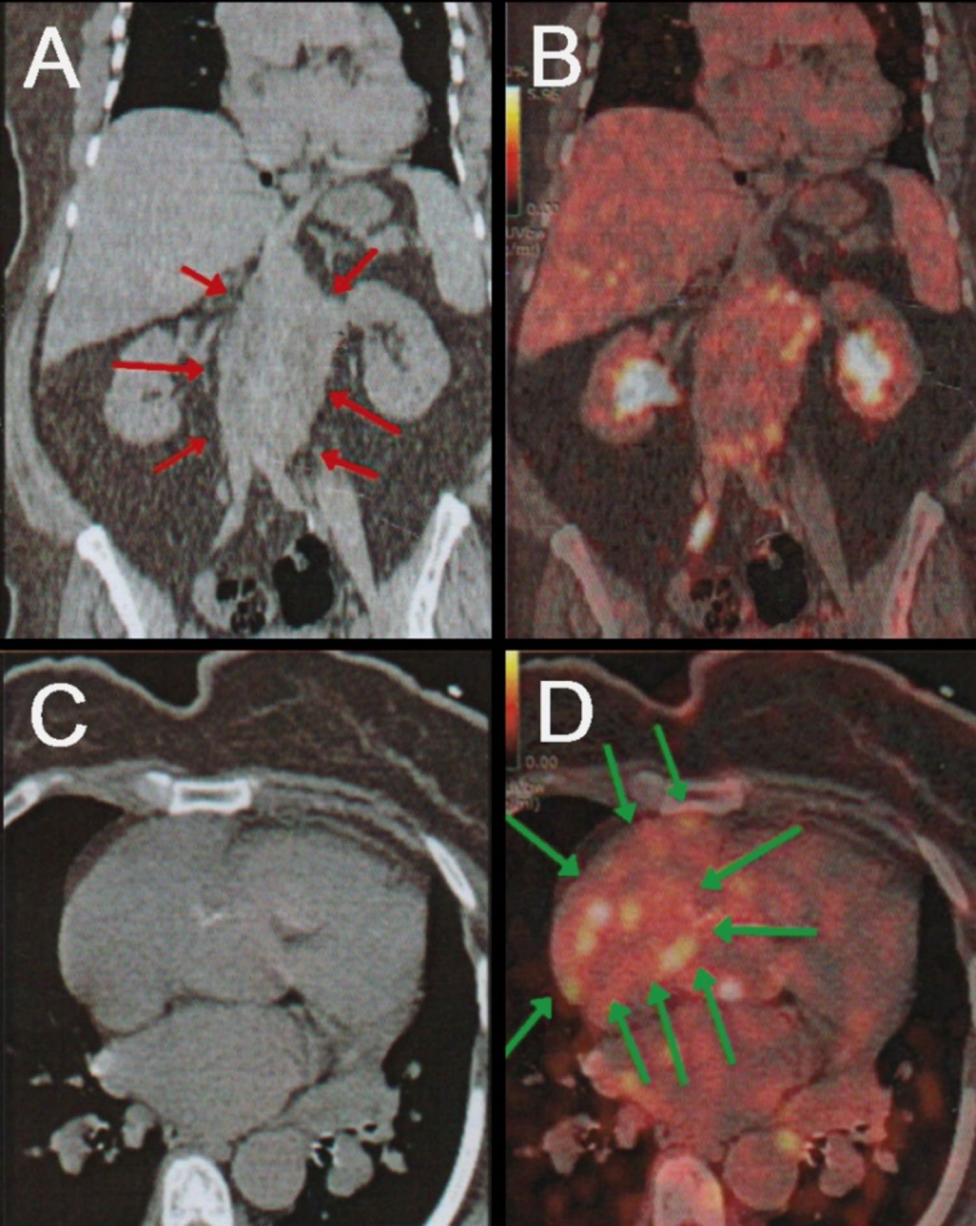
A 48-year-old female with IgG4-related disease. A Coronal non-contrast-enhanced computerized tomography (CT) and B coronal 18 fluorodeoxyglucose (FDG)-positron emission tomography (PET)/CT fusion images show 18 FDG-avid retroperitoneal soft tissue mass of amalgamated lymph nodes encasing the abdominal aorta and its branches up to the iliac bifurcation (red arrows). C Trans axial non-contrast-enhanced CT and D trans axial FDG PET/CT fusion images show 18 FDG-avid pericardial soft tissue mass compressing the right atrium (green arrows)
